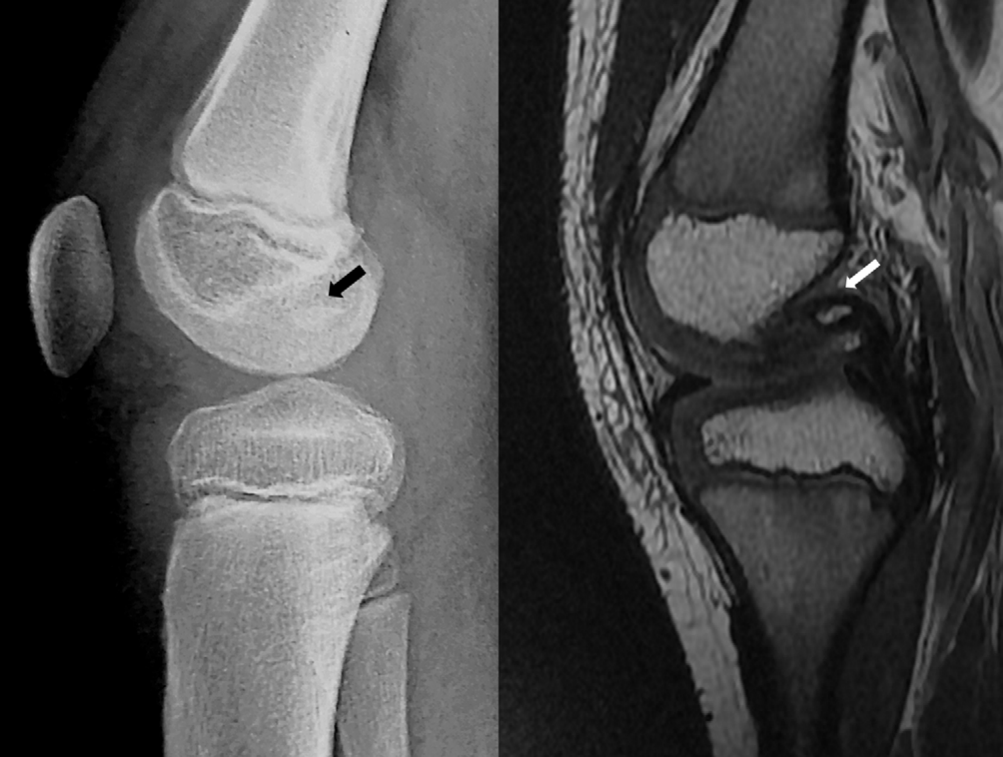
Case 2. Lateral view and sagittal sequences of second MRI showing ossification of the cartilaginous PCL avulsion on the femur.
